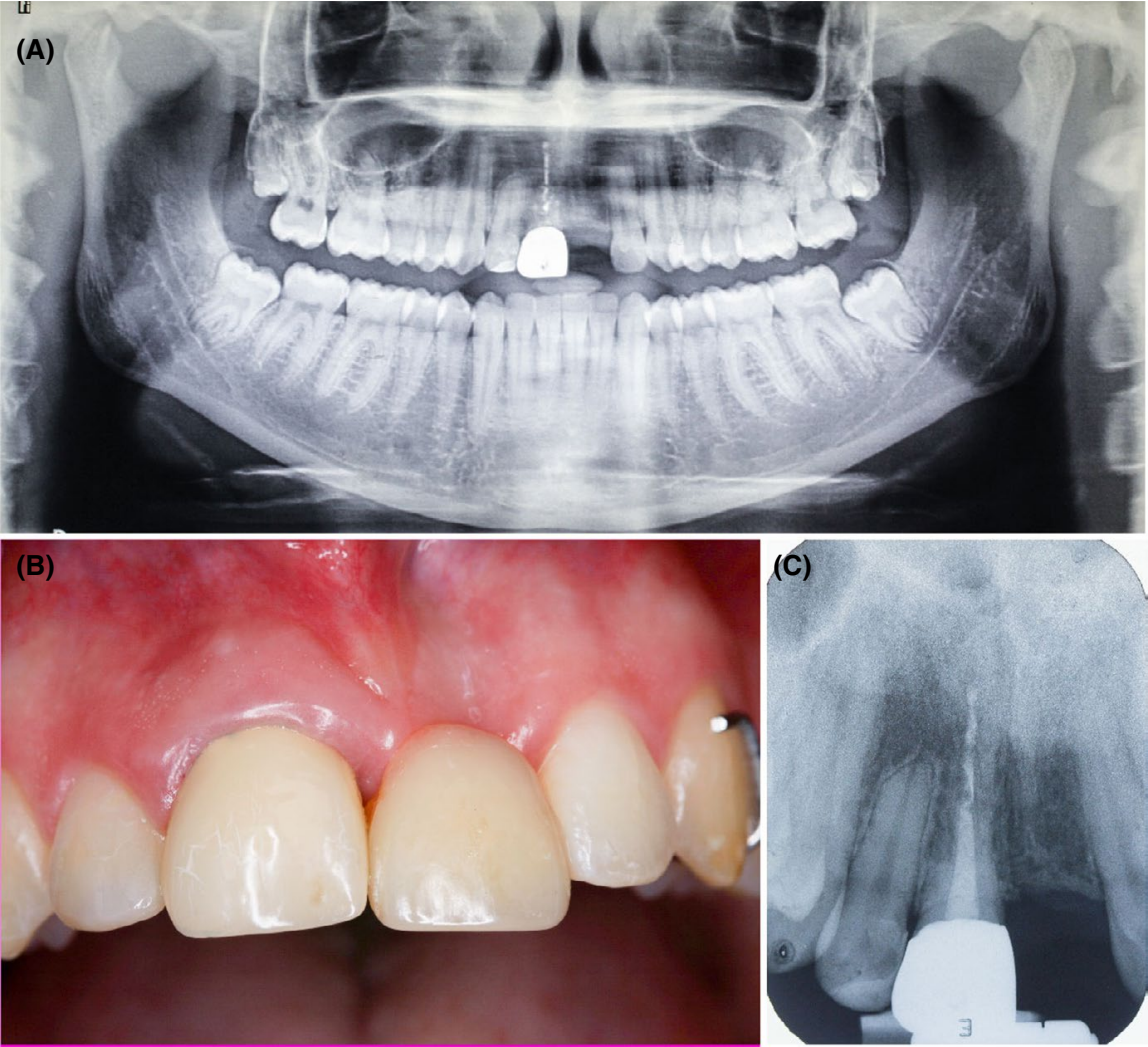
Ortopantomographic image and periapical X‐ray indicating ankylosis of tooth #11 with replacement resorption 2 years after the accident (A, C). Infra‐occlusion of tooth #11 compared to provisional restoration 2 years after trauma (B)
